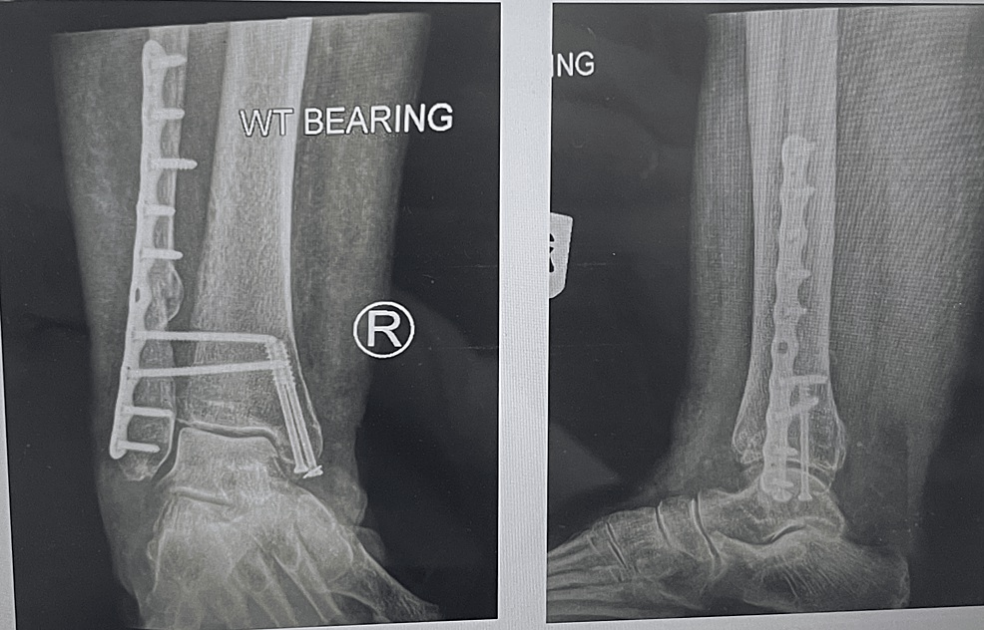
Postoperative radiograph of the bimalleolar ankle fracture with syndesmotic disruption, addressed using syndesmotic screws
